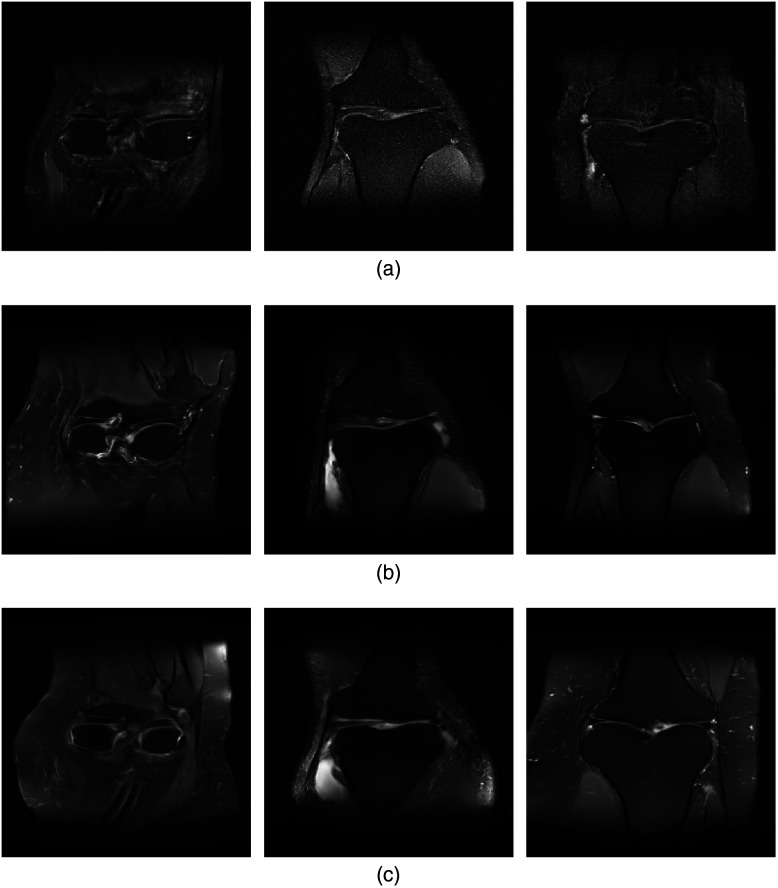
Results from emulated single-coil MRI data. (a) ProGAN-generated images. (b) ProAmGAN-generated images. (c) Sty2AmGAN-generated images.
